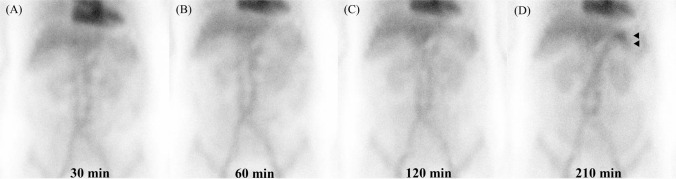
Findings of protein-losing scintigraphy. A–C Scintigraphic images obtained 30–120 min following intravenous injection of Tc-labeled tracer. D Abnormal tracer leakage from the stomach is noted at 210 min (arrow)
